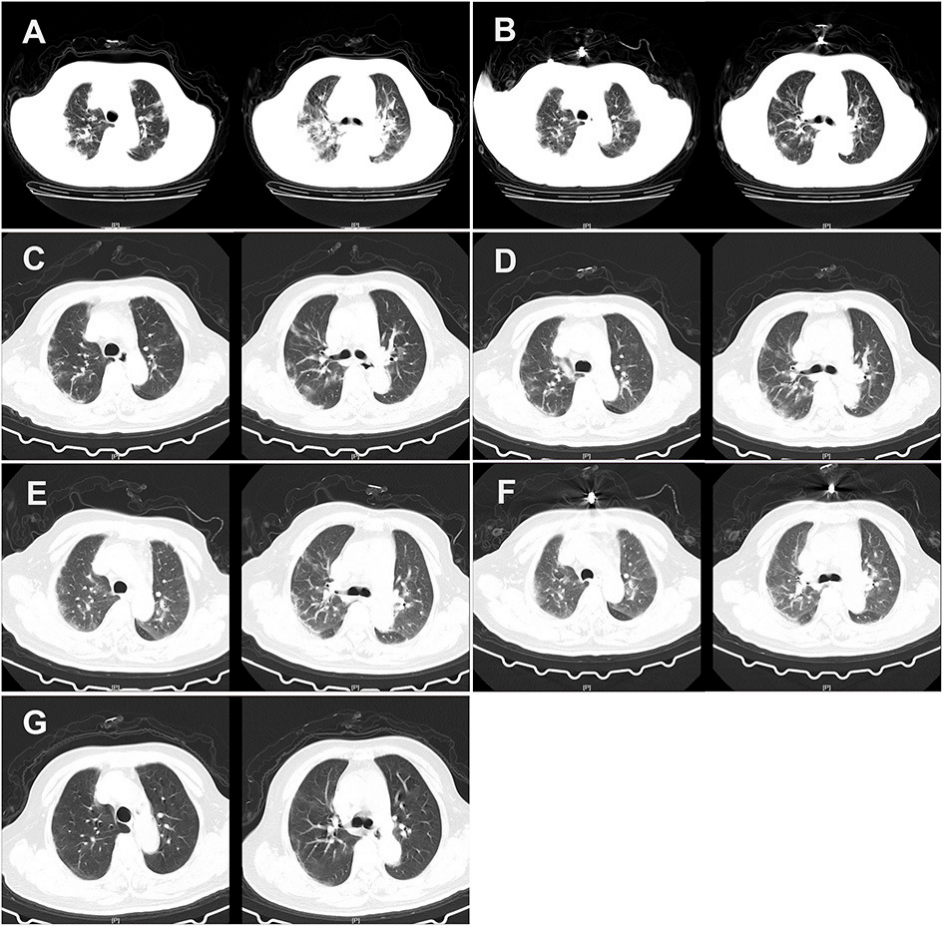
CT of the chest of the patient. (A) CT of the chest was obtained on February 12, 2020 (hospital day 2, illness day 6). The major morphogenesis of her bilateral lungs took on increased bronchovascular shadows and multiple patchy and maculas shadows, with cord-like ground-glass opacity (GGO) in the middle and lower regions of the lung. CT scan of the chest also showed increased lung markings. The texture of the trachea and blood vessels in both the lungs became thicker. (B) CT of the chest was obtained on February 16, 2020 (hospital day 6, illness day 10). The patchy lesions and maculas in both the lungs were partially absorbed. Increased lung markings were observed in the bilateral lungs. (C) CT of the chest was obtained on February 20, 2020 (hospital day 10, illness day 14). Decreased density of the patchy lesions in both the lungs was observed. The texture of the trachea and blood vessels in both the lungs became thicker. (D) CT of the chest was obtained on February 24, 2020 (hospital day 14, illness day 18). The pulmonary lesions remained unchanged. (E) CT of the chest was obtained on February 28, 2020 (hospital day 18, illness day 22). There was no obvious change in the patchy lesions in both the lungs. GGO was slightly increased. (F) CT of the chest was obtained on March 3, 2020 (hospital day 22, illness day 26). The major lesions of the bilateral lungs were not absorbed. (G) CT of the chest was obtained on March 10, 2020 (hospital day 29, illness day 33). The multiple patchy and maculas shadows of the bilateral lungs were further absorbed and the bronchovascular shadows were reduced.
